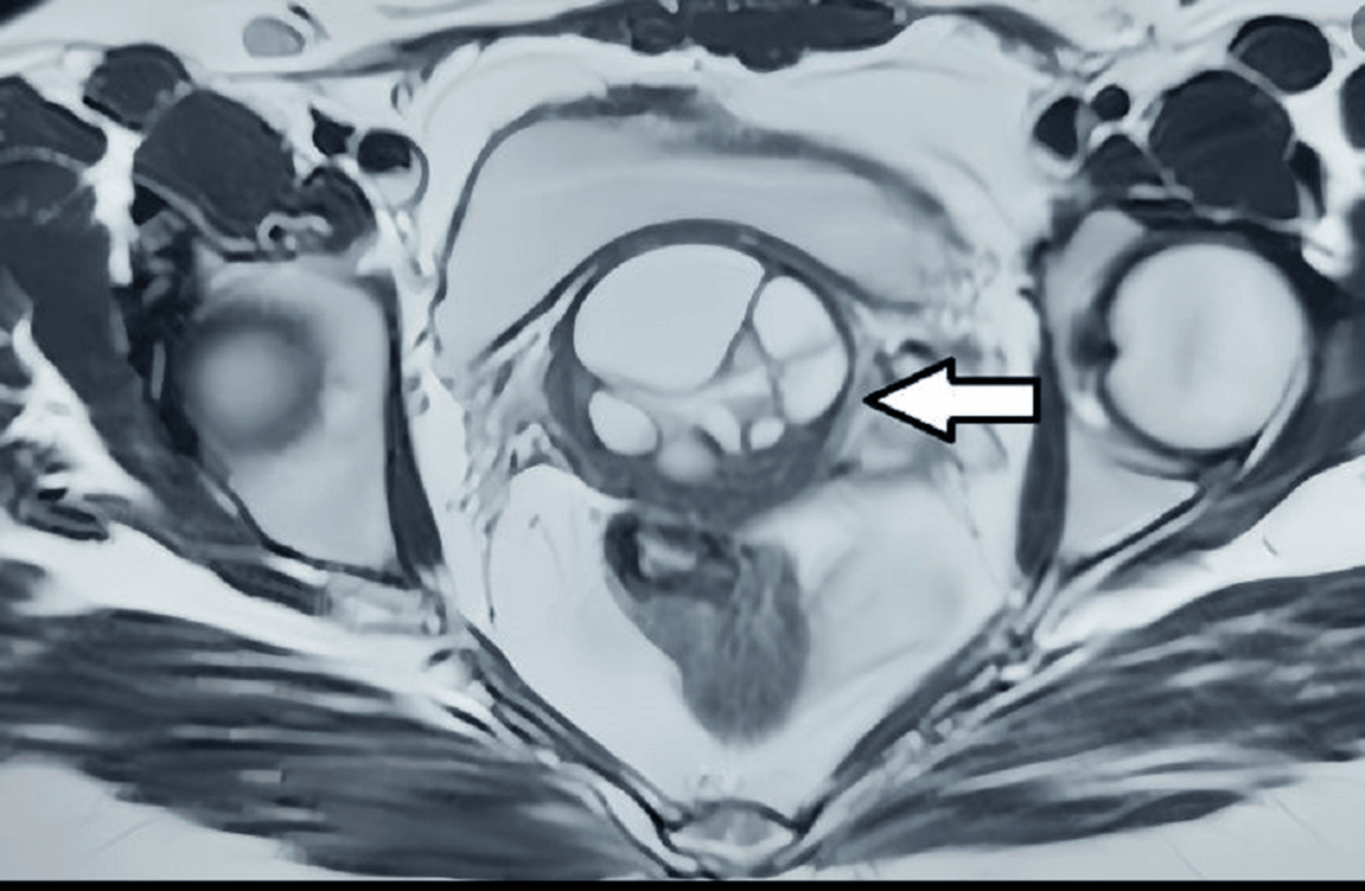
T2-weighted MRI showing the axial section of the pelvis. A hyperintense septated cystic lesion involving the cervix is noted. No involvement of the parametrium is noted.
